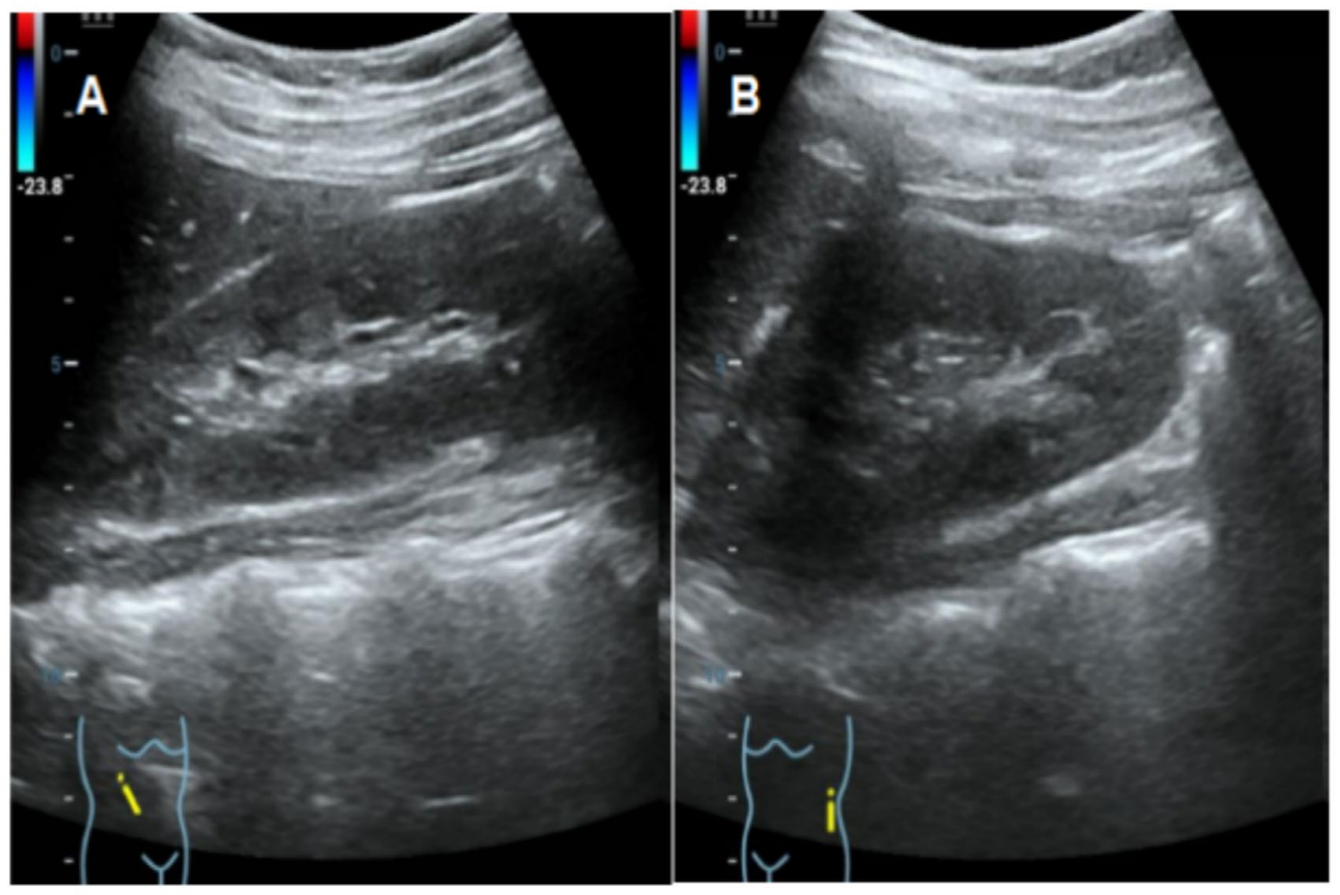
Ultrasound images of the patient’s urinary system (A: right kidney; B: left kidney).
